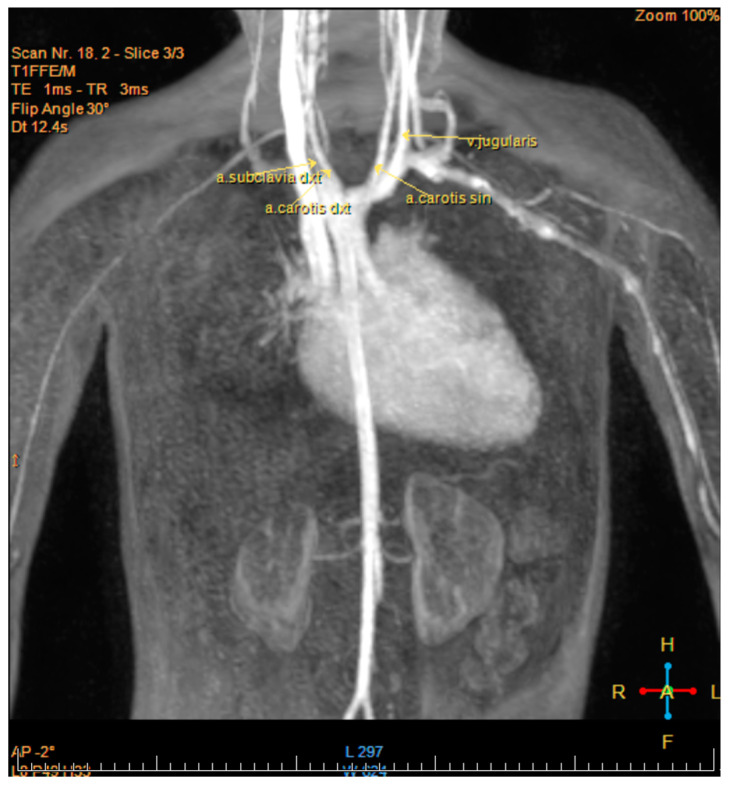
Cardiac magnetic resonance imaging (MRI) showing right aortic arch.
